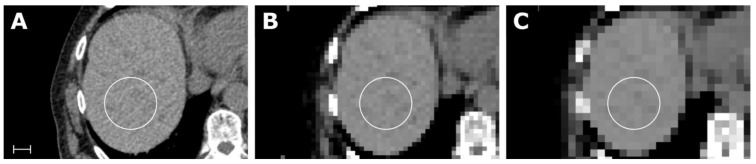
Samples of an abdominal CT (A) with corresponding maps for the feature Root Mean Squared (RMS), with a resolution of 5 × 5 × 5 mm in (B) and 10 × 10 × 10 px in (C). Scale indicates 2 cm. The value for RMS in the liver parenchyma from the conventional extraction and for both maps was 1060. Details of the scan and feature extraction as well as a full list of all values is available in electronic Table S1.
